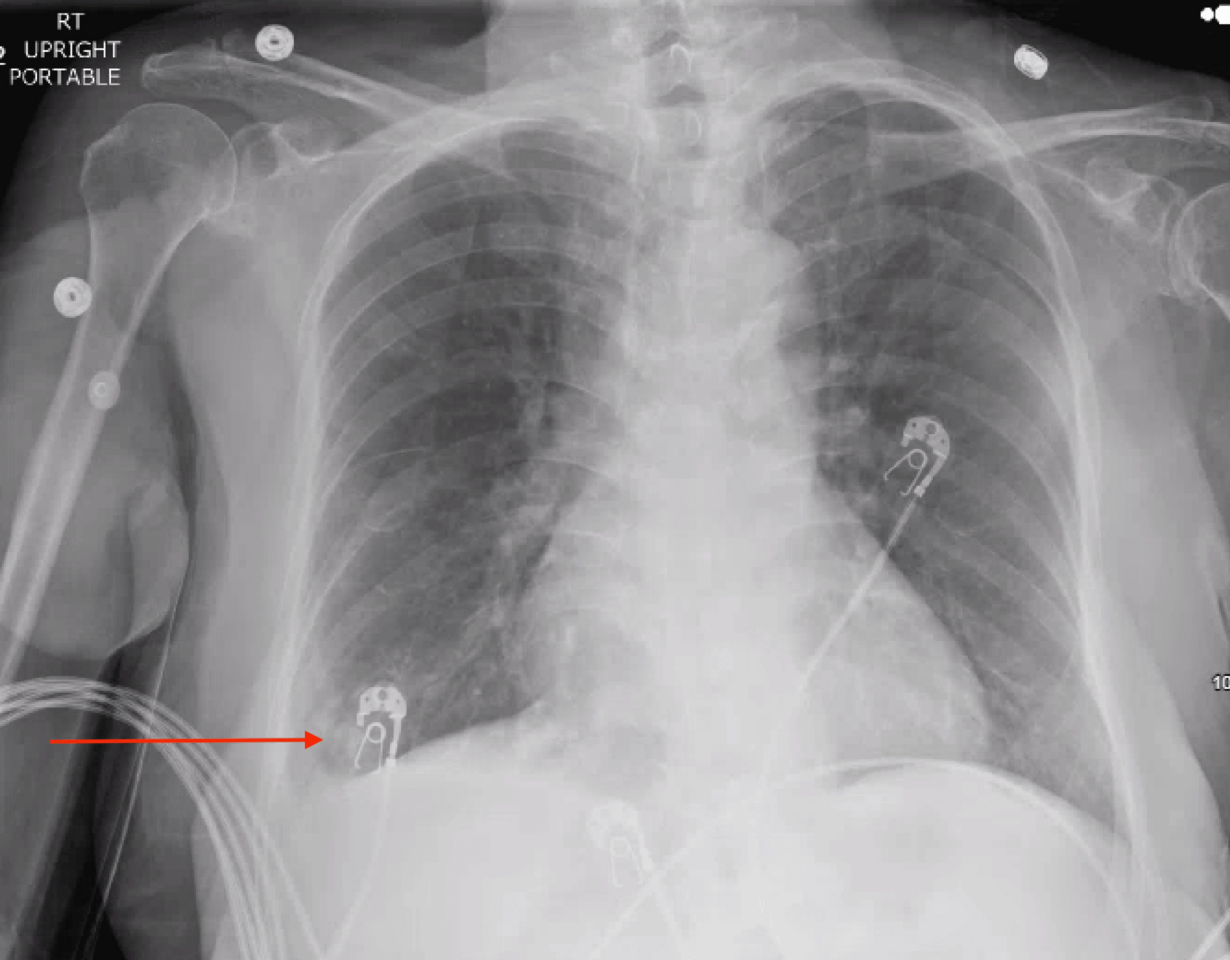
Anterior-posterior chest radiograph showing right lower lobe lung opacity with small pleural effusion
